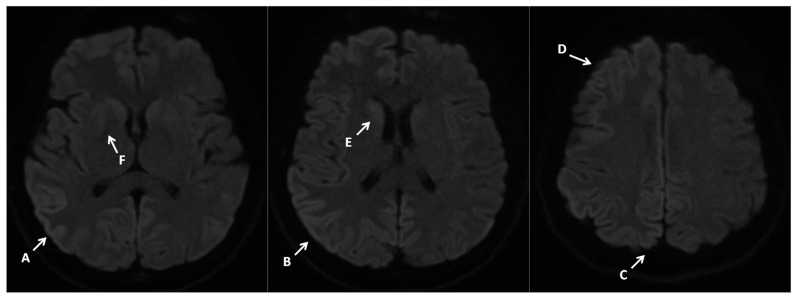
Contrast MRI imaging 5 April 2024. Imaging performed on a 1.5T scanner. Brain MRI shows abnormal imaging with increased signals on DWI imaging of the right cerebral cortex-cortical ribbon sign (A–D). Similar changes are also seen in the right caudate nucleus (E) and putamen (F) and medially in the left parietal lobe.
